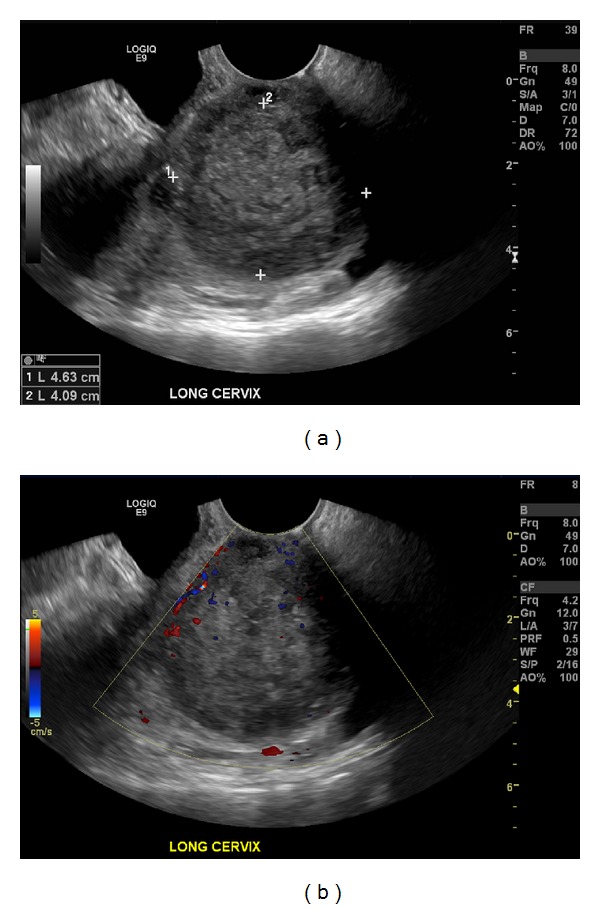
A transvaginal ultrasound. The gray-scale (a) and color Doppler images (b) demonstrate a large heterogeneously echogenic vascular mass measuring 4.6 × 4.1 × 3.6 cm, involving most of the cervix.
